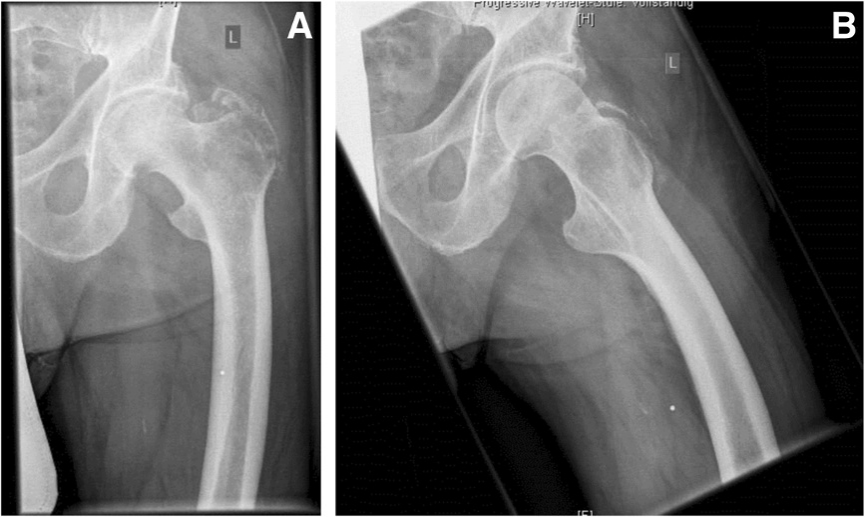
(A) and (B) Radiographs showing a representative case of a proximal femur metastasis: (A) anterior-posterior and (B) axial radiograph of the proximal femur of a 76-year-old male patient with a renal cell carcinoma metastasis. The metastasis lead to a pathological fracture of the proximal femur. An embolization has been performed pre-operatively to reduce intraoperative bleeding (coil visible in pre-operative radiographs).
